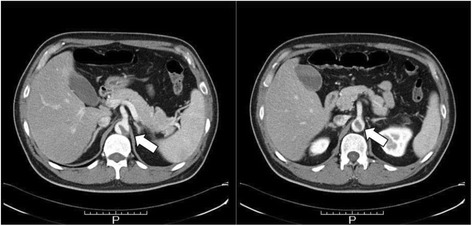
Axial view of the preoperative contrast-enhanced computed tomography. These views showed the end of the intra-aortic thrombus (*large white arrows*) indicating the likelihood of further systemic thrombosis
